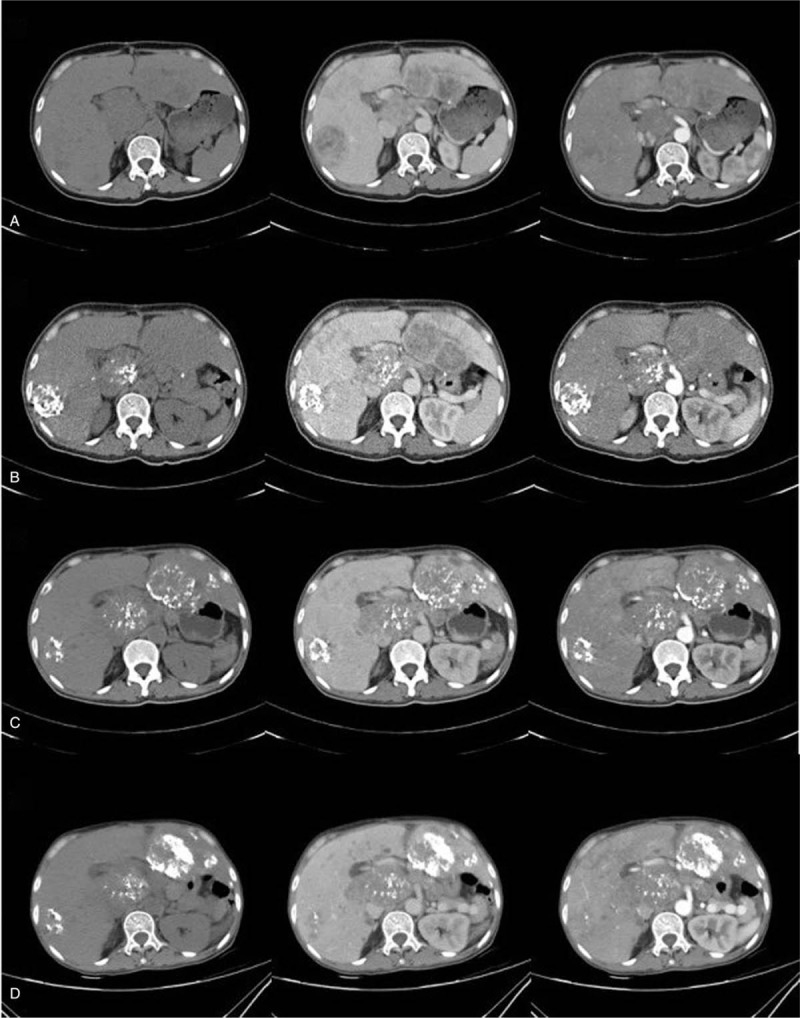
CT scan upon admission to our hospital. From left to right are plain CT scans, venous phase and arterial phase, respectively. (A) First admission, multiple lesions (maximal diameter of 55 mm) with perilesion venous and arterial enhancement. (B) Second admission, 2-month post TACE treatment. Lipiodol deposition was visible. Lesion on segment V and VIII regressed to 35 mm in diameter. Clinically assessed as SD. (C) Third admission, 2-month post 2nd TACE treatment. Lesion in the right hepatic lobe further regress and lipiodol deposition was visible. Clinically assessed as SD. (D) Three-month post 3rd TACE treatment. Multiple newly developed lesions in both left and right hepatic lobe, vena cava, and portal vein compression by hepatic lesions, ascites, and bilateral pleural effusion. Clinically assessed as disease progression. CT = computed tomography, SD = stable disease, TACE = transarterial chemoembolization.
